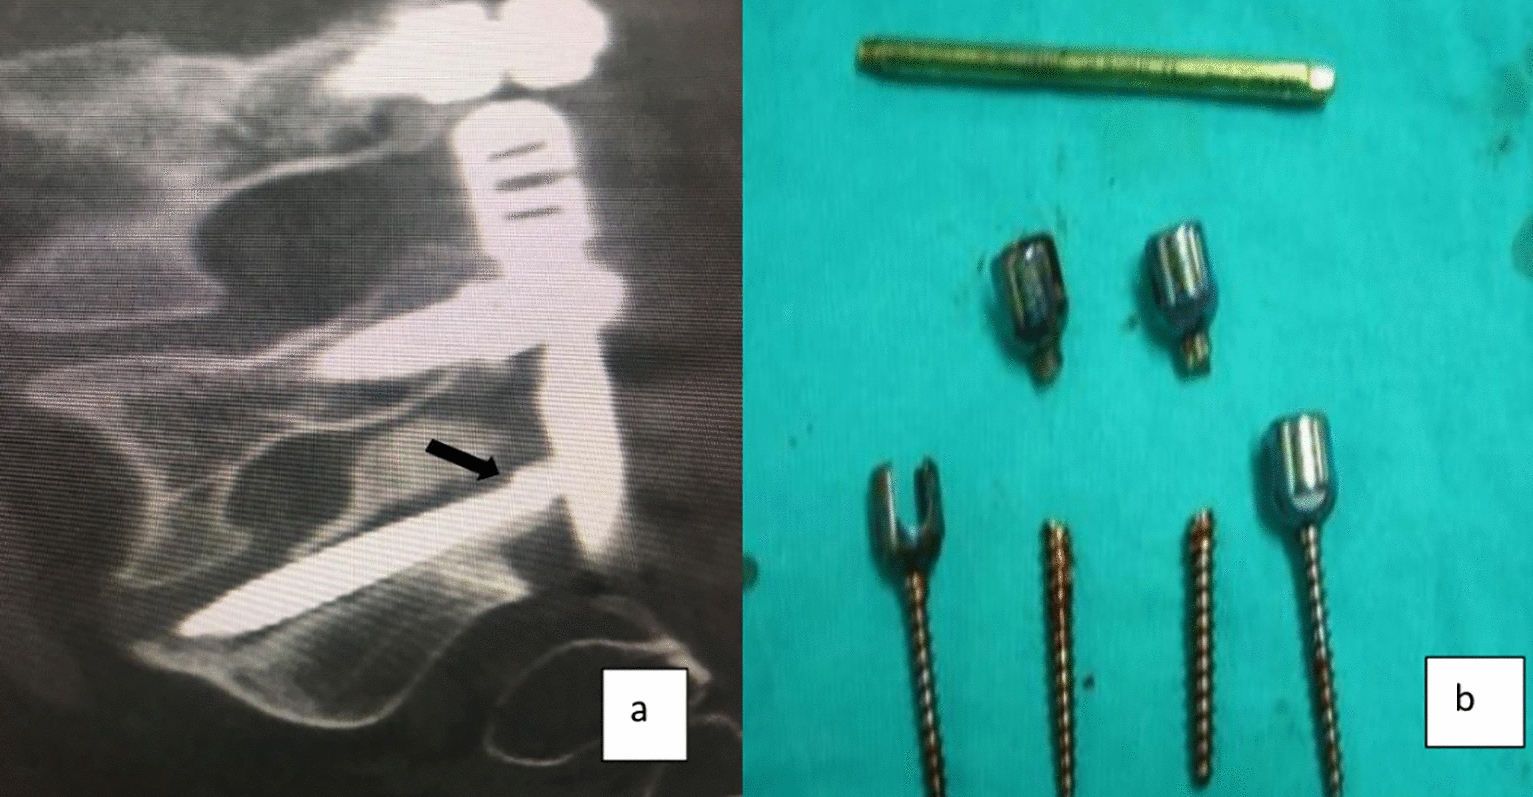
a, b Sagittal CT scans (black arrow) and image demonstrating screw breakage
